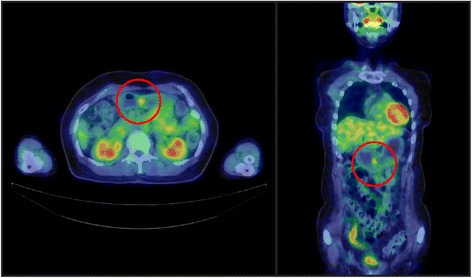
Positron emission tomography-computed tomography findings. A well-circumscribed mass was observed in the lower gastric body, with a maximum standardized uptake value of 2.7
